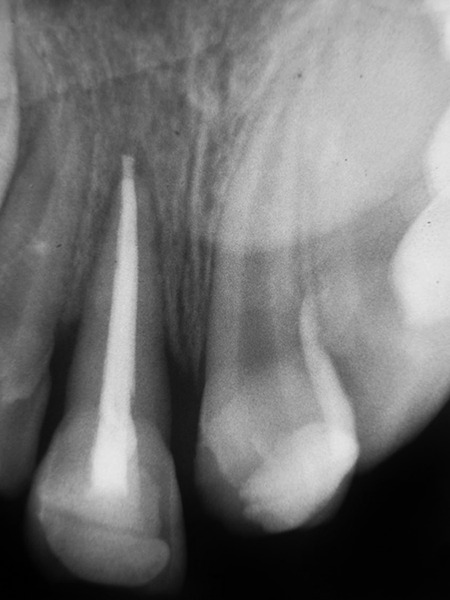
Intraoral radiograph―6 months after replantation
